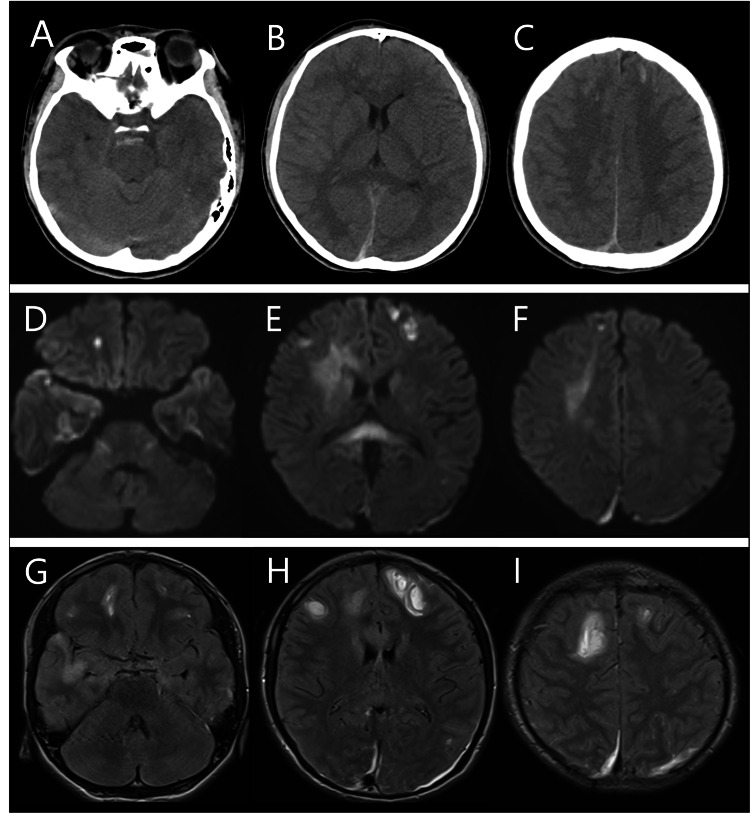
Clinical images. A-C: (Axial views) Computed tomography shows hemorrhages in the bilateral frontal lobes, basilar cisterns, and along the falx cerebri. D-I: (Axial views) Magnetic resonance image performed on the 10th day shows cerebral contusion in bilateral frontal lobes, genu and splenium of the corpus callosum on diffusion-weighted (D-F) and fluid-attenuated inversion recovery images (G-I).
